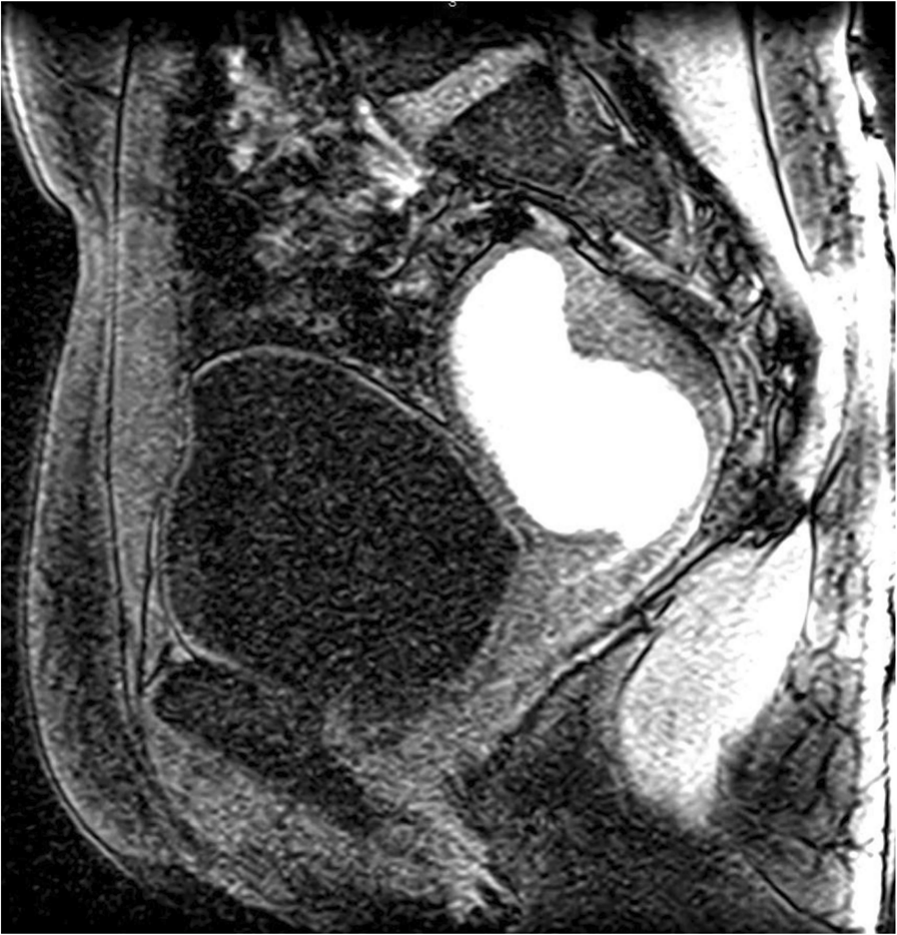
Magnetic resonance imaging. Fluid-filled endometrial cavity and endocervical canal. The canal is non-dilated at 1 to 2 mm
